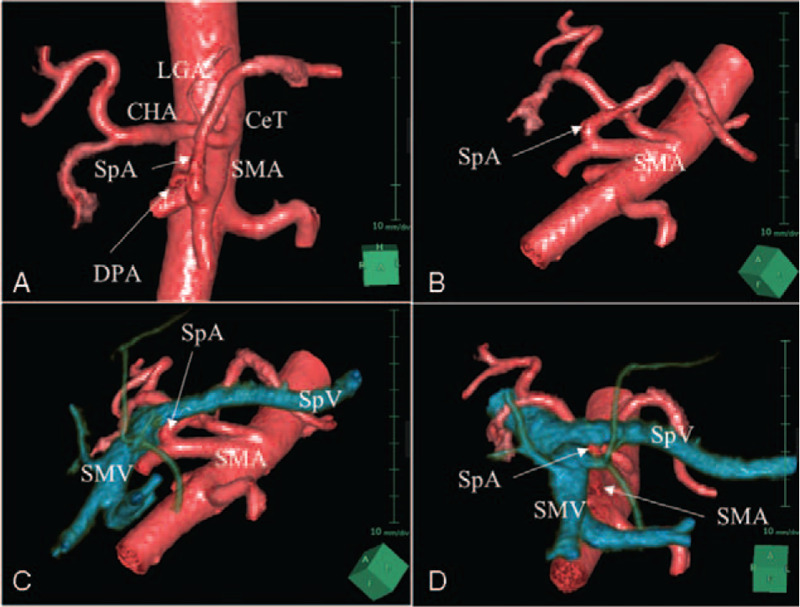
Preoperative 3D-CT images. A: CeT had only two branches: LGA and CHA. SpA was originating from the SMA with DPA from its origin. B: The anatomical relationship between SpA and SMA was revealed in a left anterolateral view. C: SpA was originating at the level of the inferior border of SpV and ascending dorsally to SpV. D: The origin of SpA was confirmed from the inferior border of the pancreas. *CeT* = celiac trunk, *CHA* = common hepatic artery, *DPA* = dorsal pancreatic artery*, LGA* = left gastric artery*, SpA* = splenic artery, *SpV* = splenic vein.
